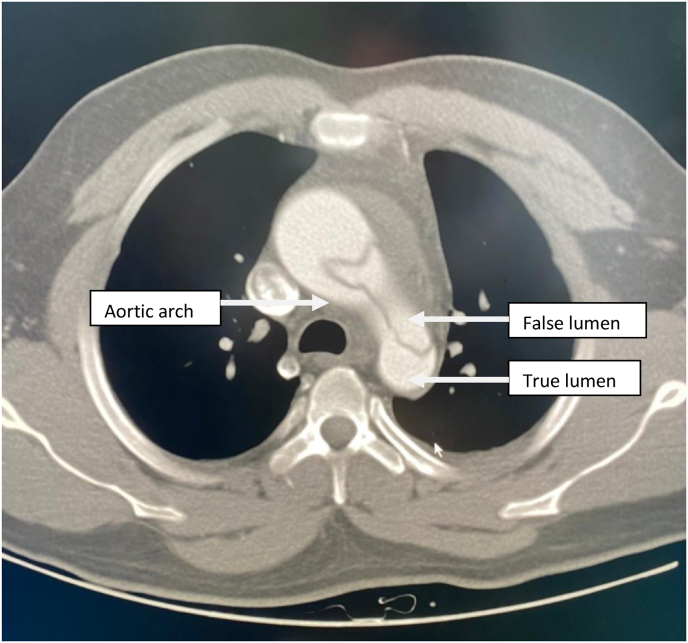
a CT scan image showing the dissection in the aortic arch (c) with the true (b) and the false lumen (a).
